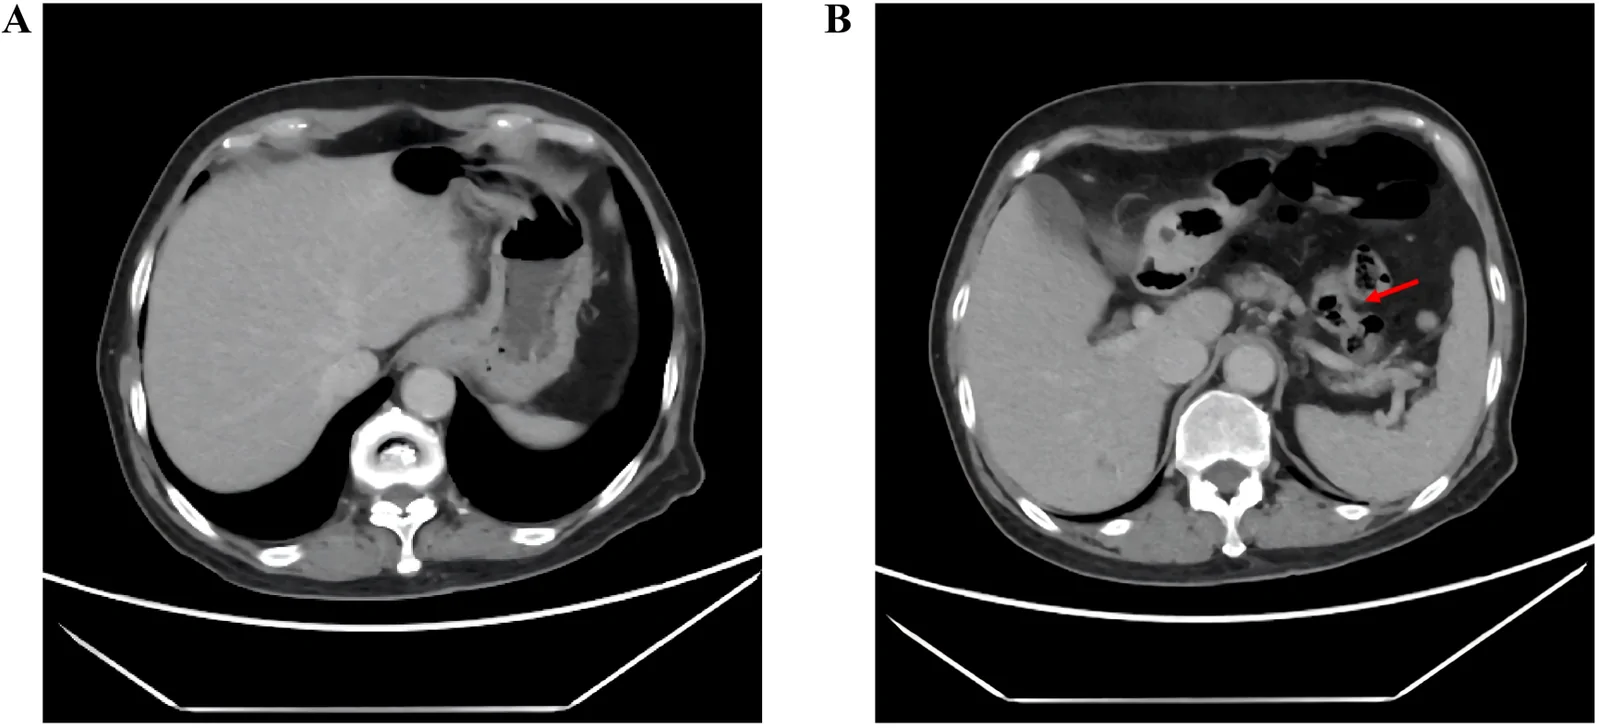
The enhanced abdominal CT showed: (A) The wall of the lower end of the esophagus, cardia, and stomach is thick, with a diameter of about 4.32cm. (B) Multiple enlarged lymph nodes were seen around the stomach, and the short diameter of the large ones was about 1.2cm.
